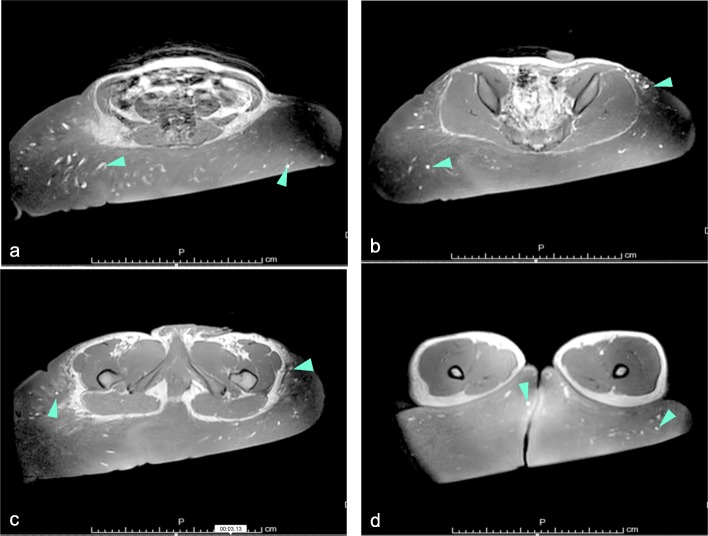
T2 weighted images (T2WI) of the patient’s lesion before surgery. a .Superior side of the lumbar area of the lesion. b. Buttocks and sacral area of the lesion. c. Upper thigh area of the lesion. d. Lower thigh area of the lesion. The plexiform neurofibroma shows relatively high intensity in T2WI of MRI. There are many high intensity signal lesions in the images (green arrow head). These are considered to be dilated veins or arteries
